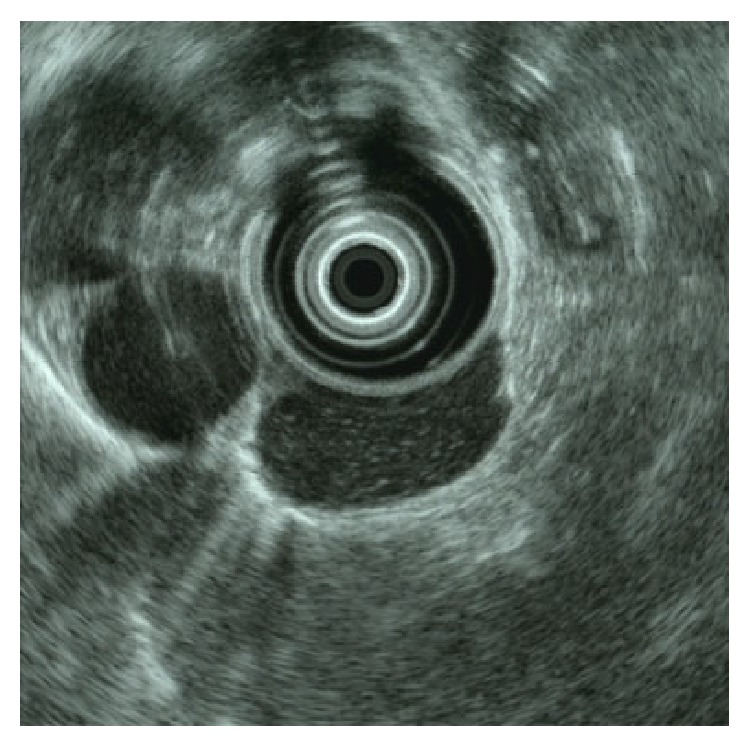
Endoscopic ultrasonography. The image shows a cystic mass extending from the lower esophagus to the cardiac region of the stomach and is filled with viscous components. No echoic debris indicative of bleeding or solid components was observed.
